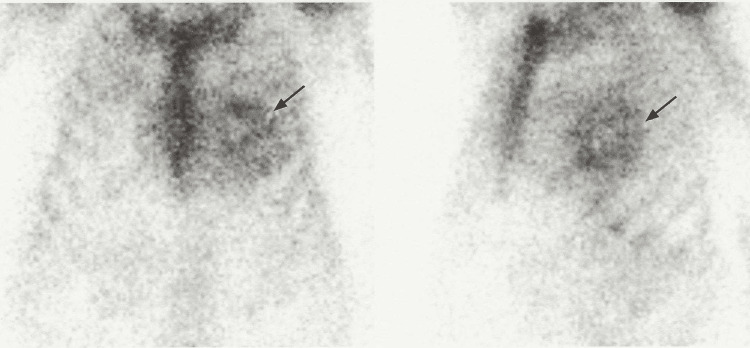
Technetium pyrophosphate scintigraphy with increased uptake of radiotracer (black arrow) within the heart and similar to bone (Grade 2)
